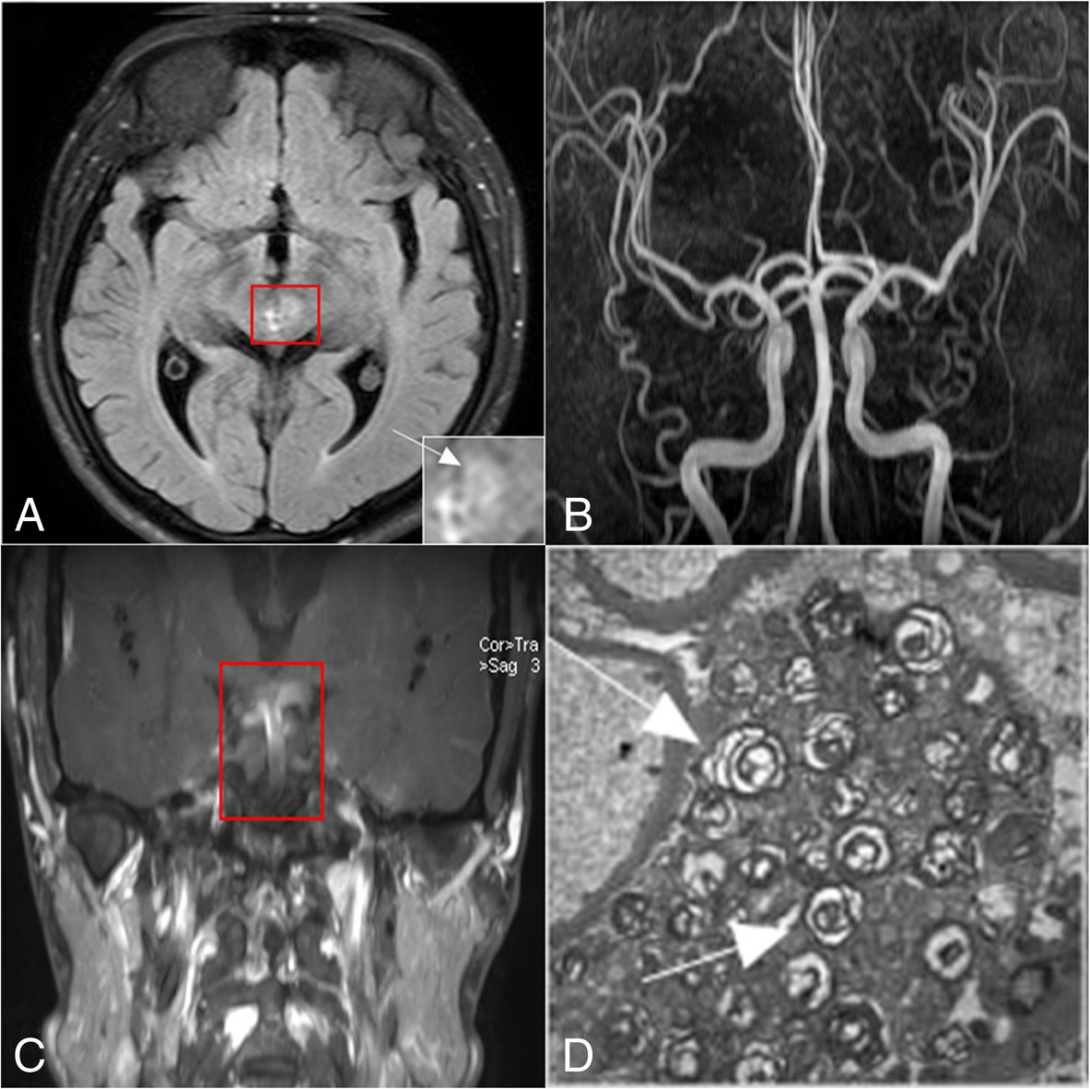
a: MR-FLAIR image of the brain with abnormal signals in the left oculomotor nucleus. b: No obvious abnormality in 3D-TOF-MRA. c: The black-blood sequence image with partial thickening and mild enhancement of the basilar artery and bilateral posterior cerebral artery. d: Kidney pathological biopsy: a large number of myeloid bodies and zebra bodies (arrow mark)
